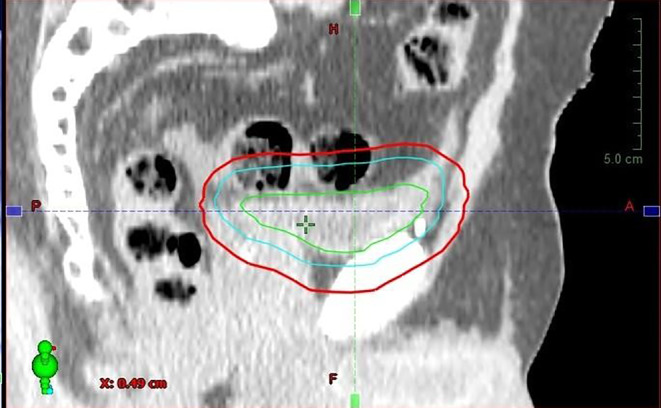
PTV reduction for one patient. *Green *bladder contour (CBCT 1), *blue *oART PTV, *red* non-ART PTV
